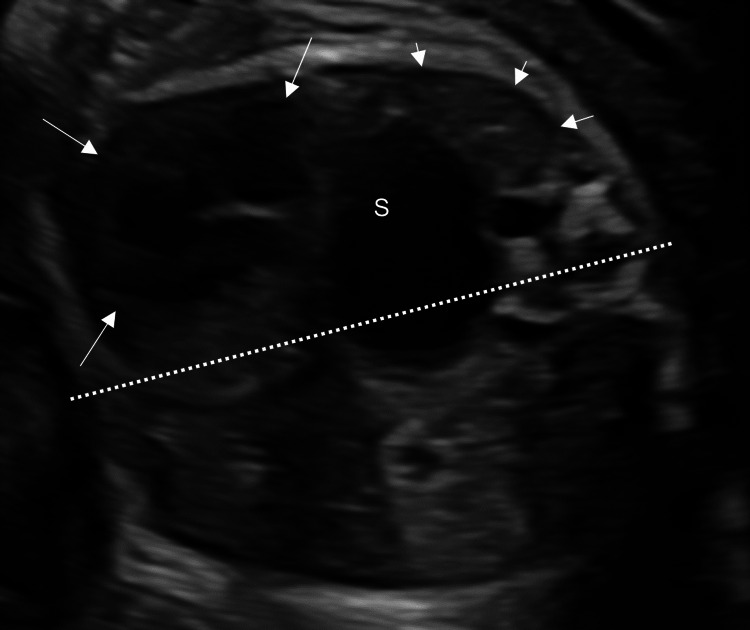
The 2D sonographic image of the fetal chest (horizontal section) Congenital diaphragmatic hernia with herniation of the stomach (S) more than halfway past the mid-line (dotted line). The heart (arrows) was shifted into the right thoracic cavity. The right lung was compressed and shrinking (arrowheads).
